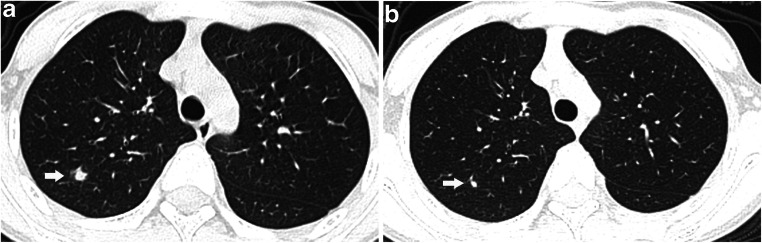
A 10-year-old boy with COVID-19, with fever and a history of exposure. a Axial thin-section non-contrast CT shows nodule shadow in the posterior segment of the right upper lobe (*arrow*). b Follow-up CT image shows the lesion is markedly reduced after 21 days (*arrow*)
